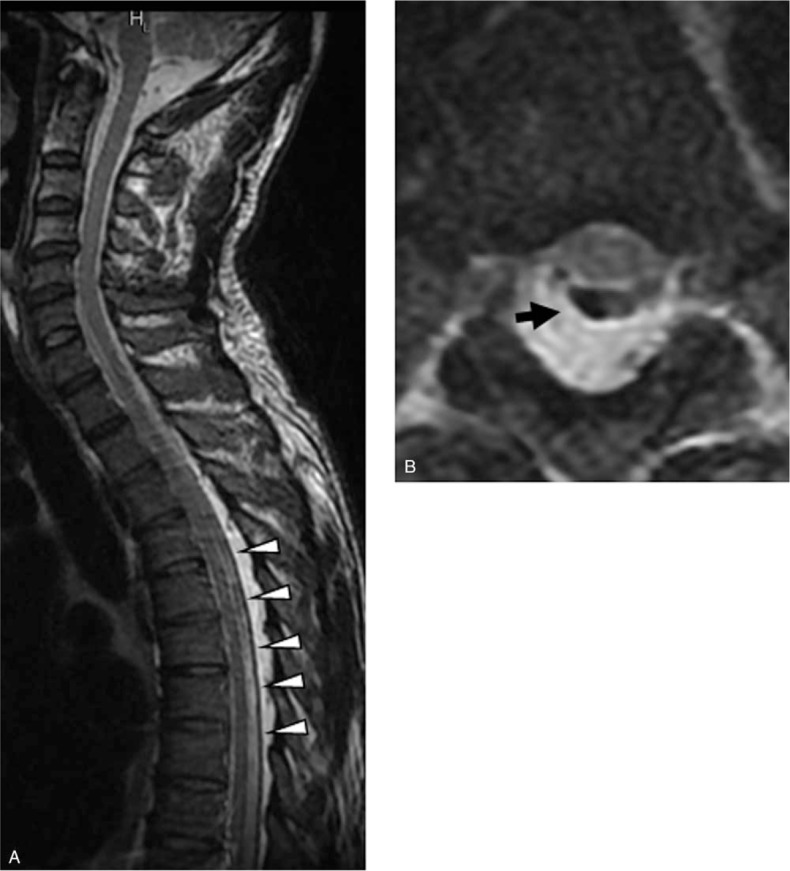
Magnetic resonance imaging of the patient's father. (A) Fibrous epidural ligament (white arrowhead) with dorsal epidural fat tissue deposition. (B) The abnormal ligament observed on axial image (black arrow).
